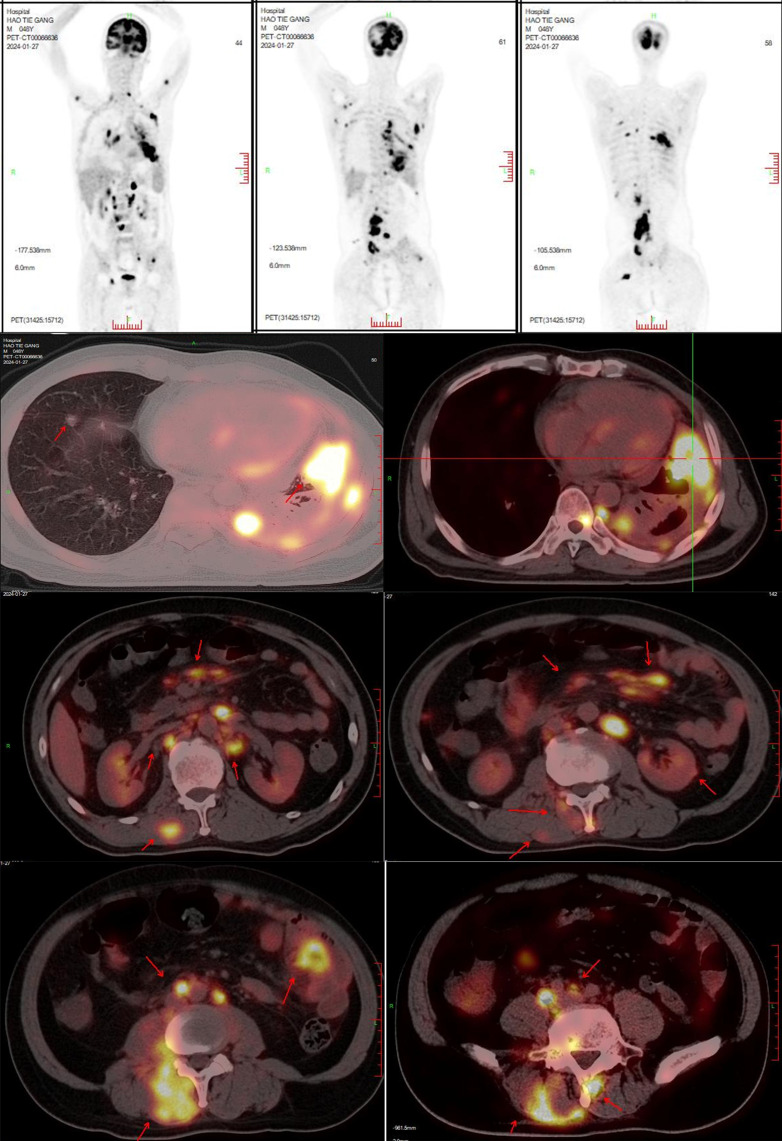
PET-CT on January 27, 2024: 1. Post-treatment findings related to lung cancer:Hypermetabolic lesion resembling residual tumor in the lingular segment of the left upper lobe, involving adjacent pleura. Cancerous lymphangitis in the left upper lobe.Multiple hypermetabolic nodules in both lungs, suggestive of multifocal pulmonary metastases.Uneven thickening of the left pleura with increased glucose metabolism, indicative of pleural metastasis.Left-sided pleural effusion. 2. Multiple hypermetabolic enlarged lymph nodes throughout the body, indicative of multifocal lymph node metastases. 3. Multiple hypermetabolic nodular lesions in the liver, suggestive of multiple hepatic metastases. 4. Bilateral adrenal gland enlargement with increased glucose metabolism, likely representing bilateral adrenal metastases. Multiple hypermetabolic nodular/mass lesions in both kidneys, suggestive of multifocal renal metastases. 5. Multiple nodular and streaky hypermetabolic lesions in the pelvic and abdominal cavities, suggestive of peritoneal metastasis with some lesions involving adjacent intestines. 6. Multiple hypermetabolic nodular/mass lesions between muscles and subcutaneously throughout the body, suggestive of multifocal metastatic lesions. 7. Multiple hypermetabolic lesions indicative of widespread bone destruction, likely representing multiple bone metastases. 8. Hypermetabolic nodular lesion in the right temporal and occipital lobe, suggestive of brain metastasis, accompanied by perilesional edema. 9. Left lower lung lobe collapse with inflammation, and pericardial effusion.
